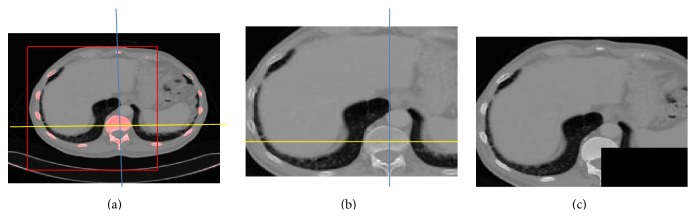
ROI extraction from 3D-CT data volume.
